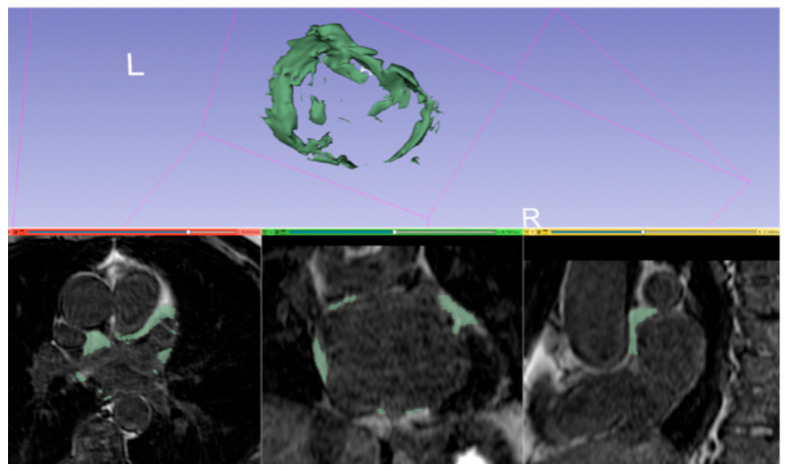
Example of segmentation and 3D reconstruction of LA EAT at cardiac MRI. L: left; R: right.
